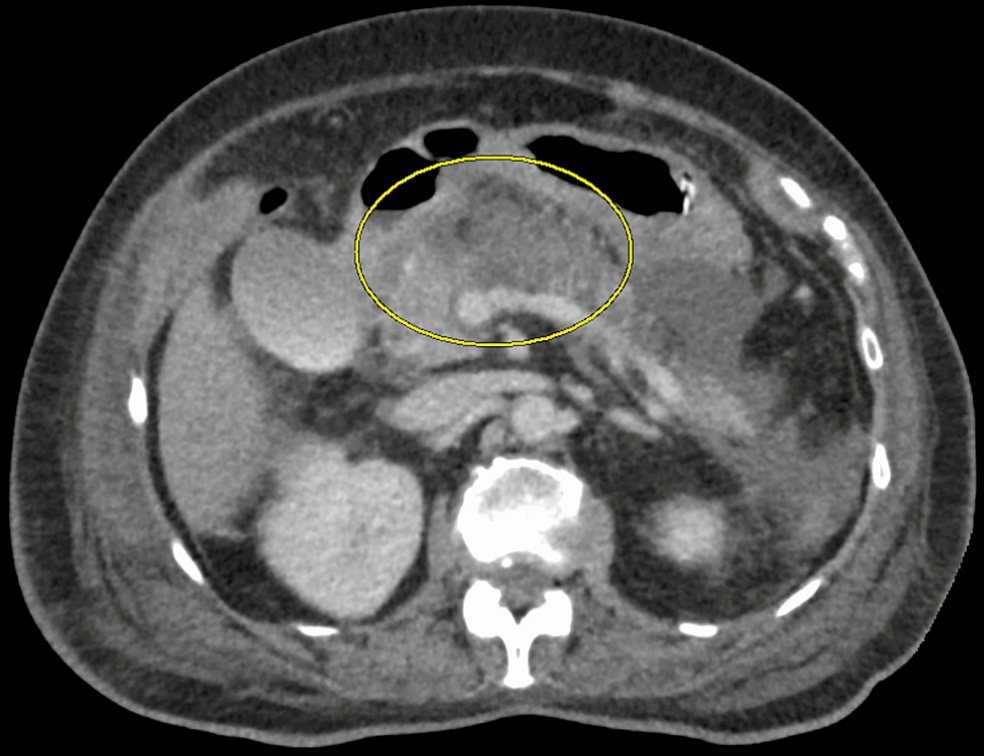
Repeat CT suspects worsening pancreatitis with possible necrosis (circled).
